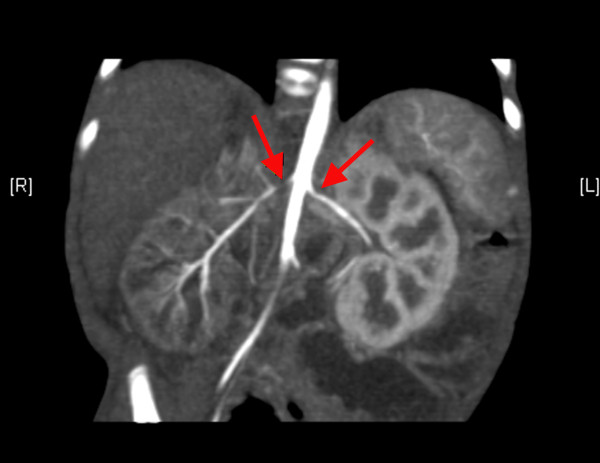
A computed tomography angiogram shows stenosis of both renal arteries near the ostium (arrows).
